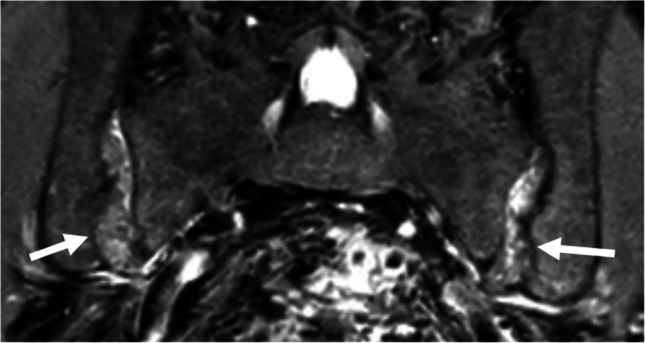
A 31-year-old man with chronic kidney disease. Extensive subchondral bone resorption on the iliac side of both SIJs (arrows) without adjacent BME or sclerosis on coronal FS T2-weighted image
